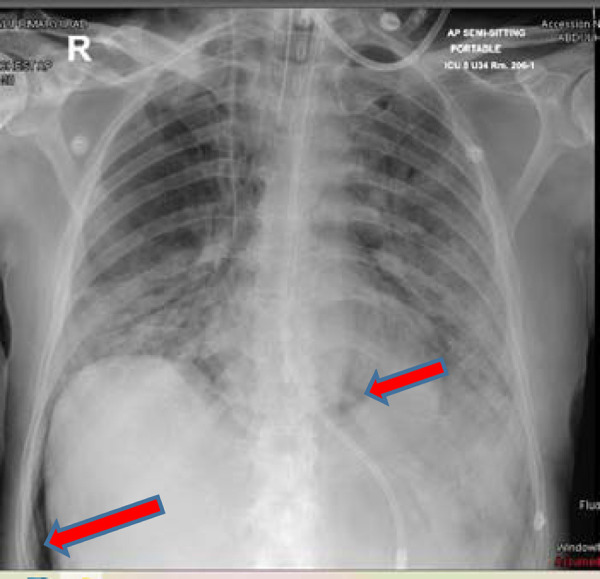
Chest X-ray image showing pneumomediastinum and pneumoperitoneum
